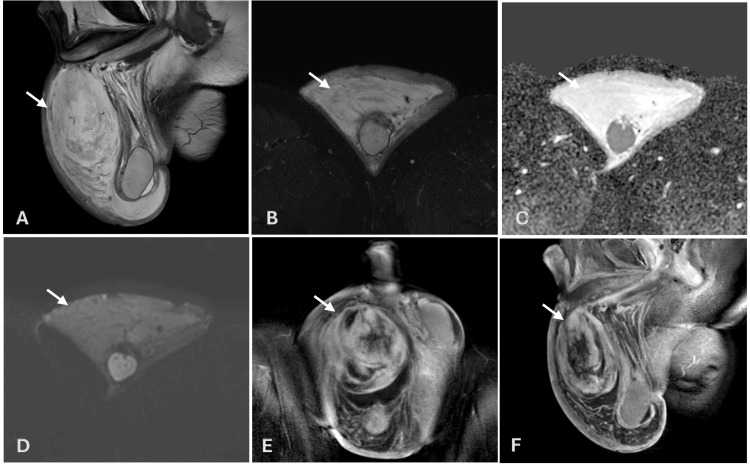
Scrotal MRI (A) T2-weighted image demonstrates a hyperintense extratesticular lesion (white arrow) with no significant signal drop on the T2 fat-saturated sequence (B). Diffusion-weighted imaging (C) and the corresponding apparent diffusion coefficient map (D) show no evidence of diffusion restriction within the lesion. Postcontrast images (E, F) demonstrate heterogeneous enhancement of the lesion.
